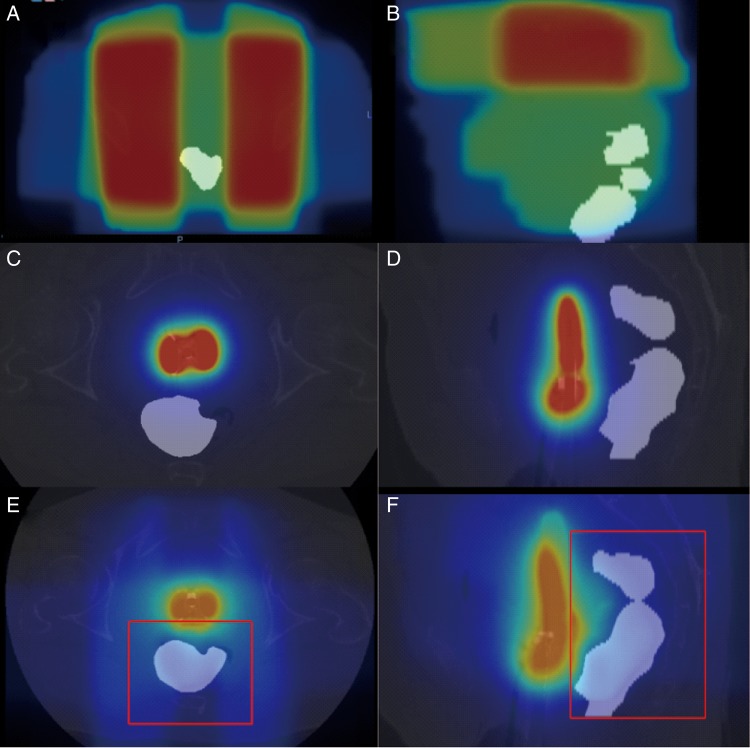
The typical dose distribution projected to CT images before and after DIR. (A) The dose distribution of EBRT before DIR from axial view. To make it easy to see, CT values within a rectal contour and tandem/ovoid applicator and outside them were replaced using in-house software; (B) sagittal view. (C) The dose distribution of ICBT before DIR from the axial view; (D) sagittal view. (E) The cumulative dose distribution after DIR. An area surrounded by the red line displayed a rectangular parallelepiped volume of interest that included the rectal contours. Abbreviations: CT = computed tomography, DIR = deformable image registration, EBRT = external beam radiotherapy, ICBT = intracavitary brachytherapy.
